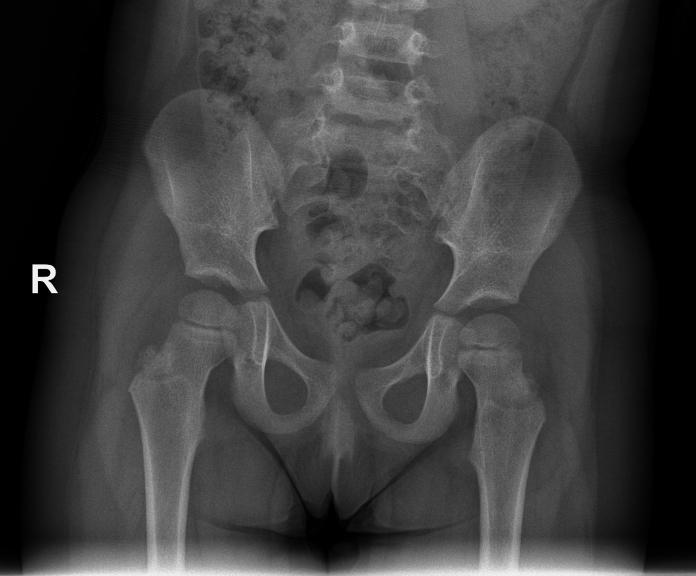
Pelvis X-ray at 8-month follow-up.
